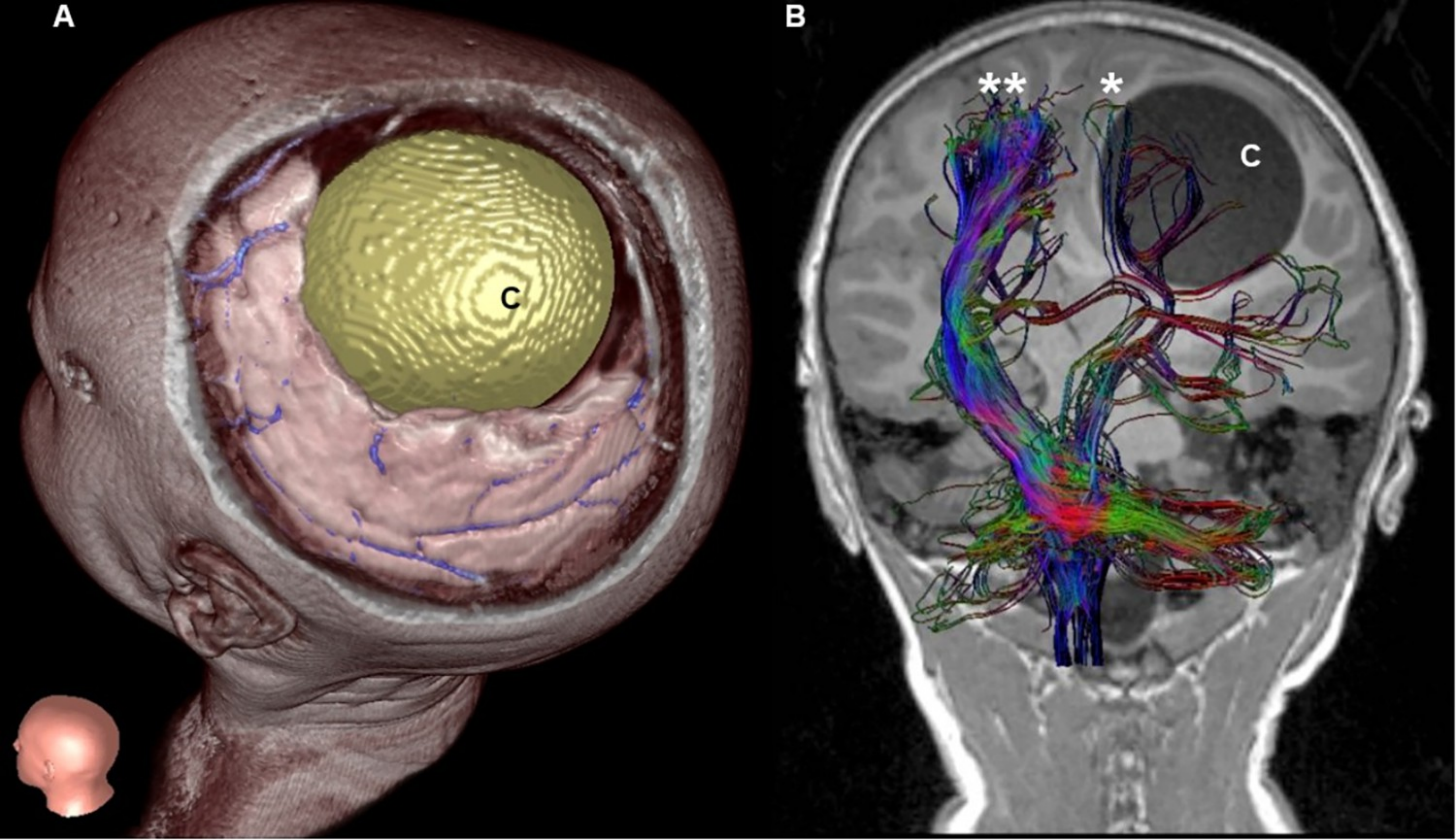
A) Tridimensional reconstruction of the left frontal-parietal cystic lesion determining massive brain compression. B) T1 weighted Magnetic Resonance imaging, coronal section integrated by diffusion tensor imaging and 3D fiber tract reconstruction. The cystic lesion (C) compression on the left cortical-spinal tract (*) is shown in comparison to the controlateral cortical-spinal tract ().
